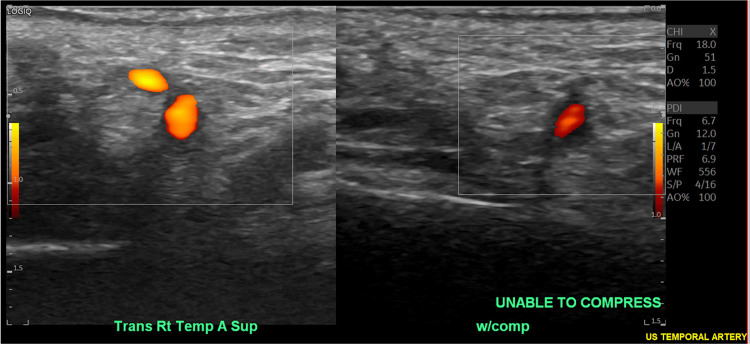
Temporal artery ultrasound revealing lack of compressibility of the right temporal artery (compression in right frame with notable continued arterial flow).
